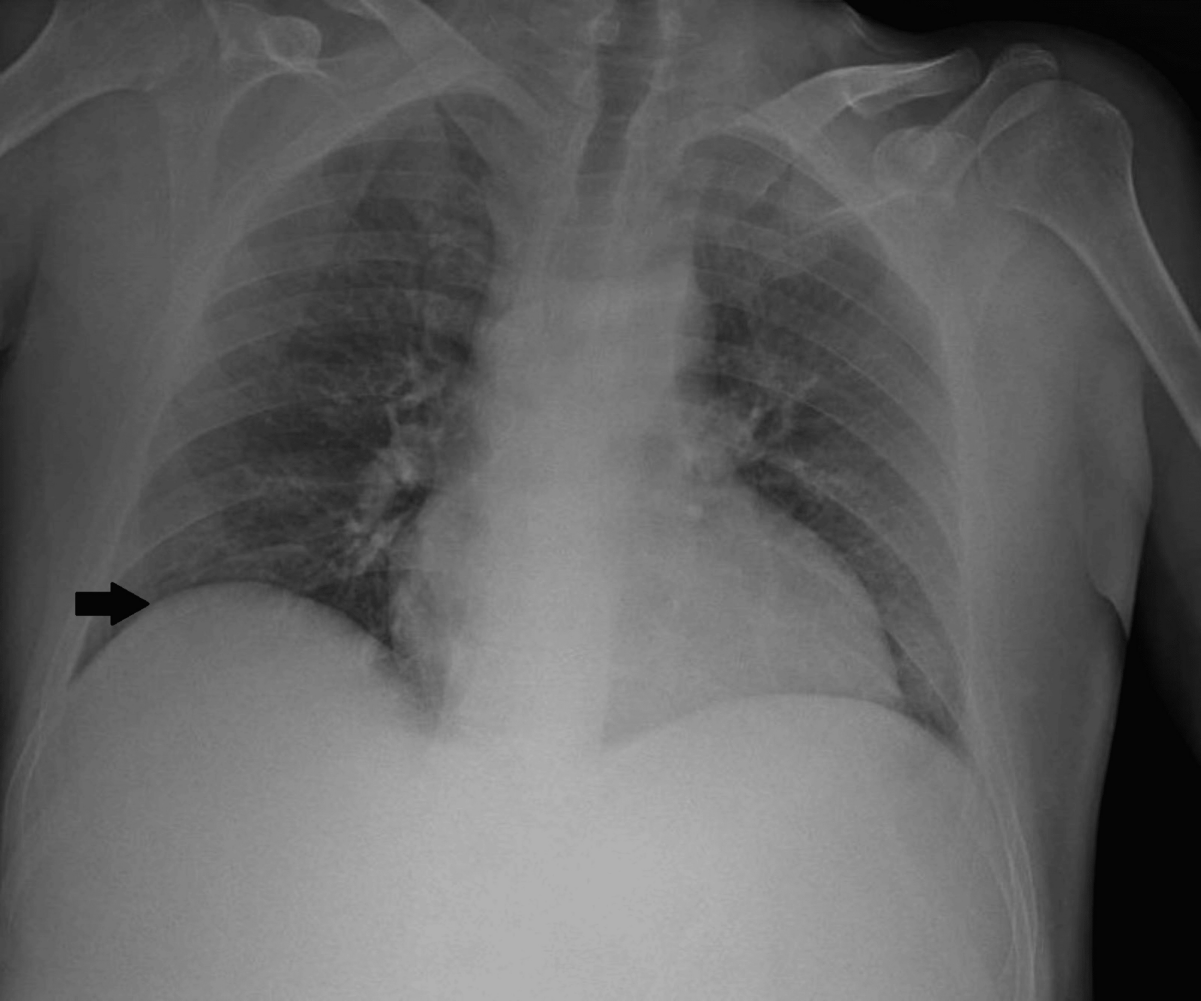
Chest X-ray with a slight elevation of the right hemidiaphragm (black arrow) and an enlarged cardiothoracic ratio
